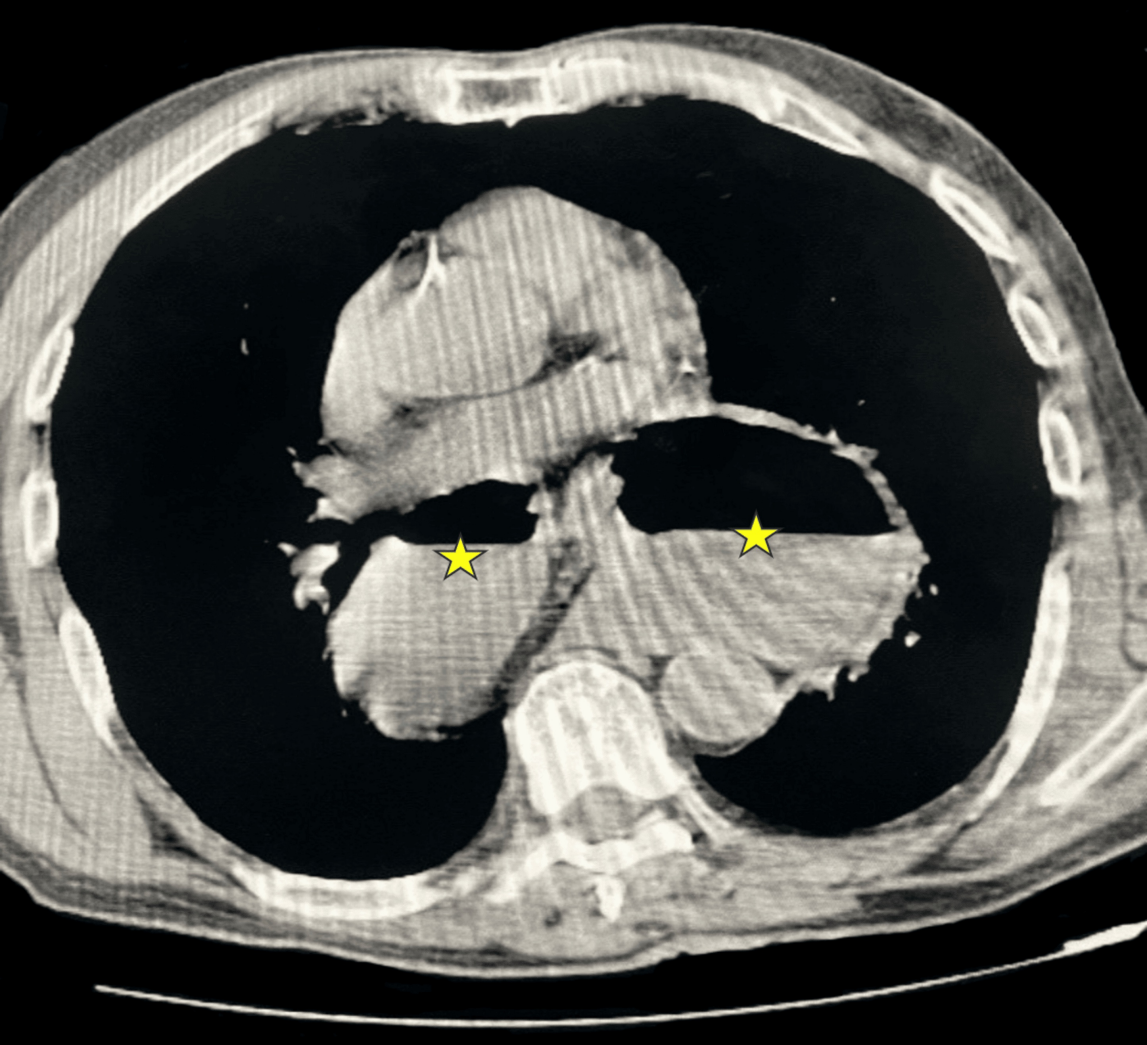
Axial thoracic computed tomography Shows a markedly distended intrathoracic stomach within the posterior mediastinum, with air-fluid levels (asterisks).
